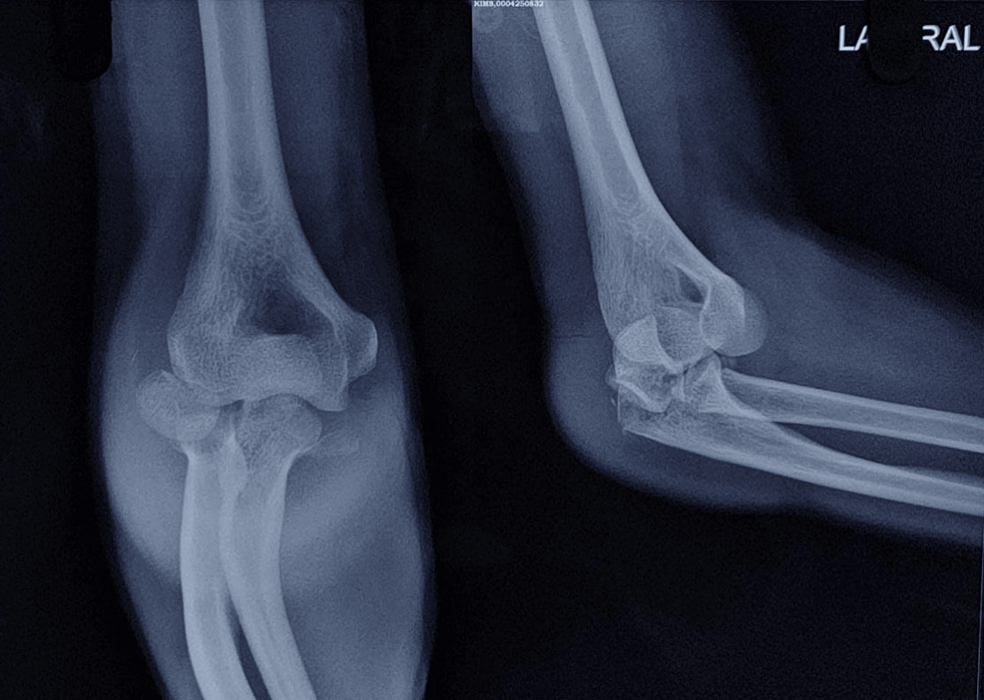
Initial pre-operative radiographs showing a right side radial head fracture associated with a comminuted fracture-dislocation of the olecranon.
